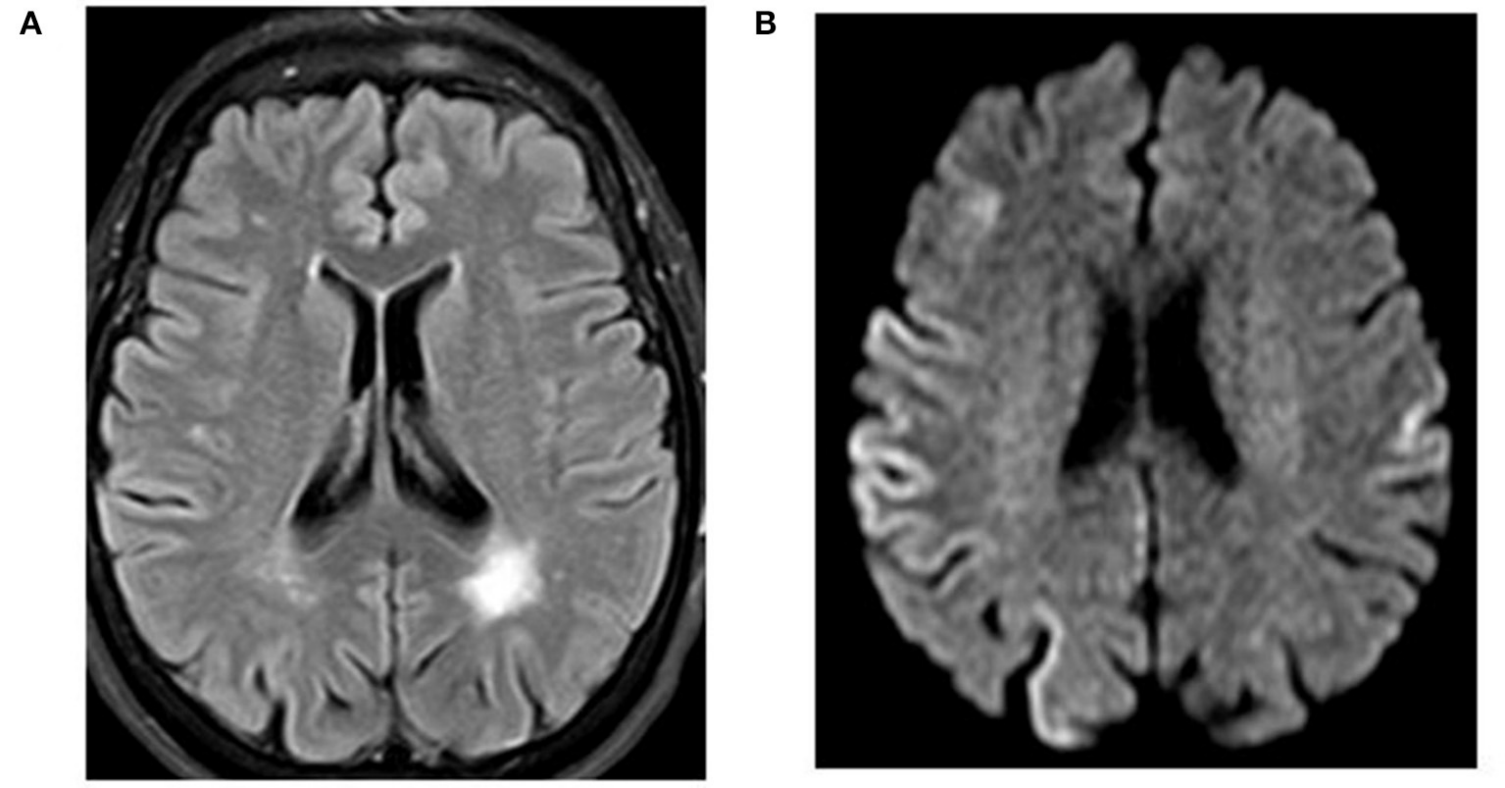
Case 23. Magnetic resonance imaging of the brain. (A) FLAIR indicating a left parietal lobe old infarction. (B) Diffusion-weighted magnetic resonance imaging with restricted diffusion at the right parietal cortex.
